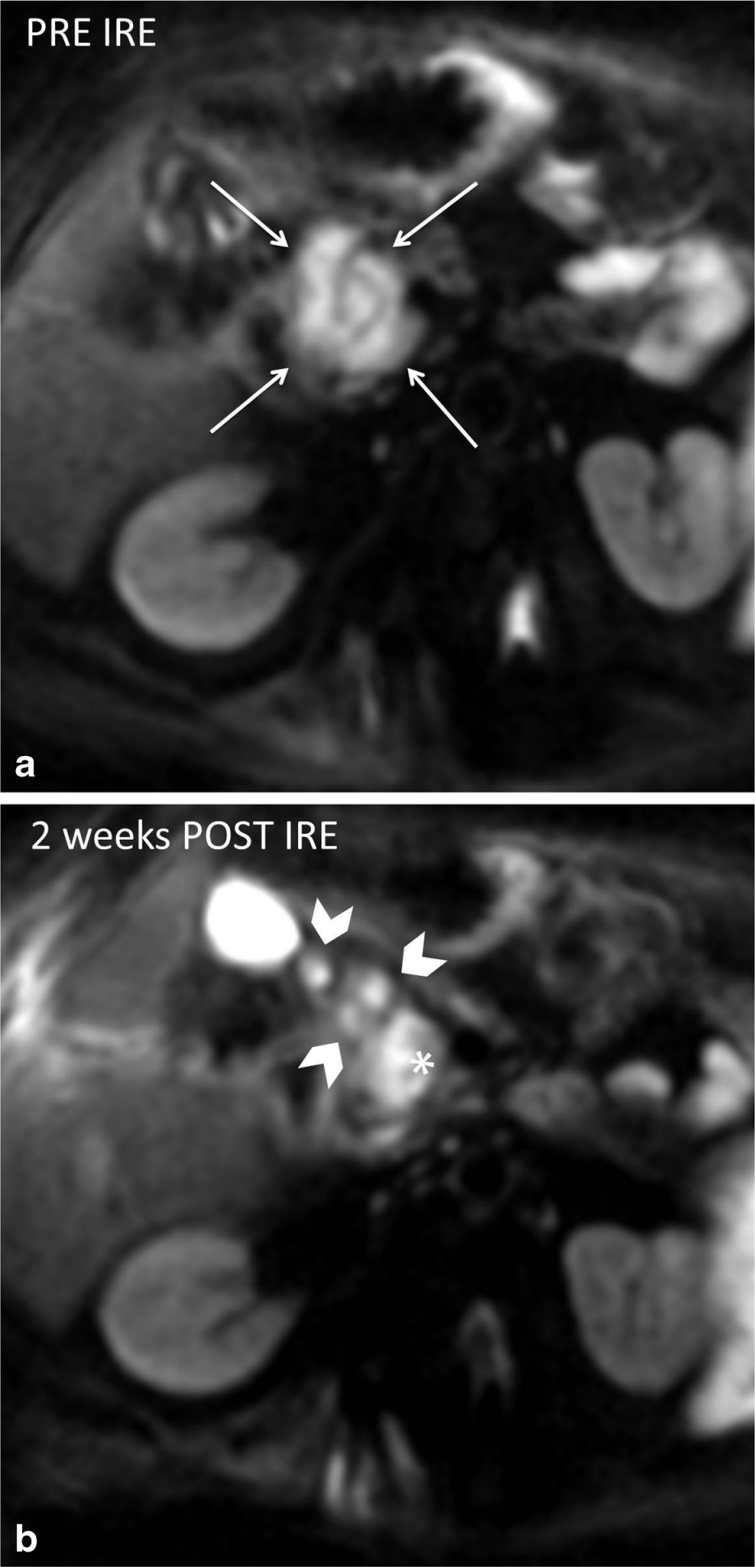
DWI b800 MR images showing (a) a hyperintensity of the tumour pre-IRE (*arrows*); (b) hypointensity of the ablated area with hyperintense marginal spots and a lymph node at 6 weeks post-IRE
